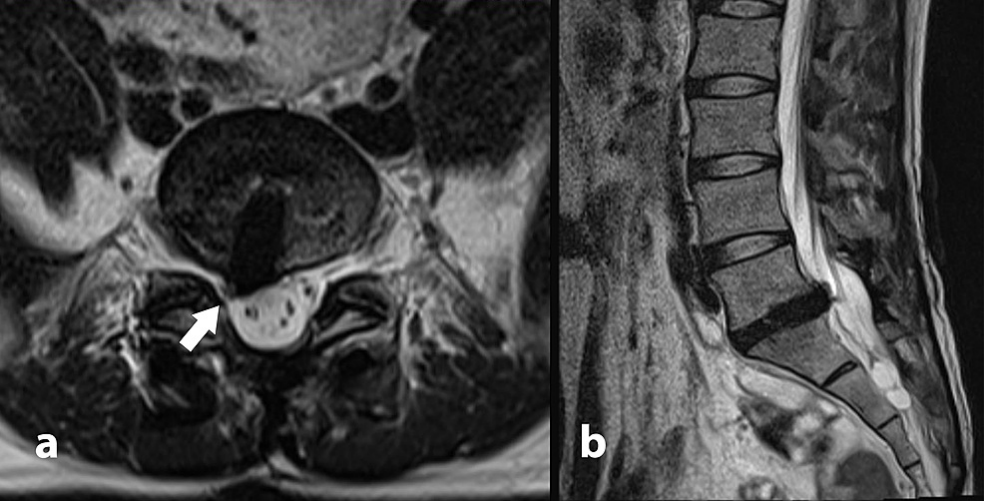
T2-weighted axial cut (a) and sagittal view (b) of MRI at L5/S1 level showing impingement of S1 traversing nerve root caused by the interbody tantalum cage. Arrow showing impingement of the tantalum cage on traversing S1 nerve root.
